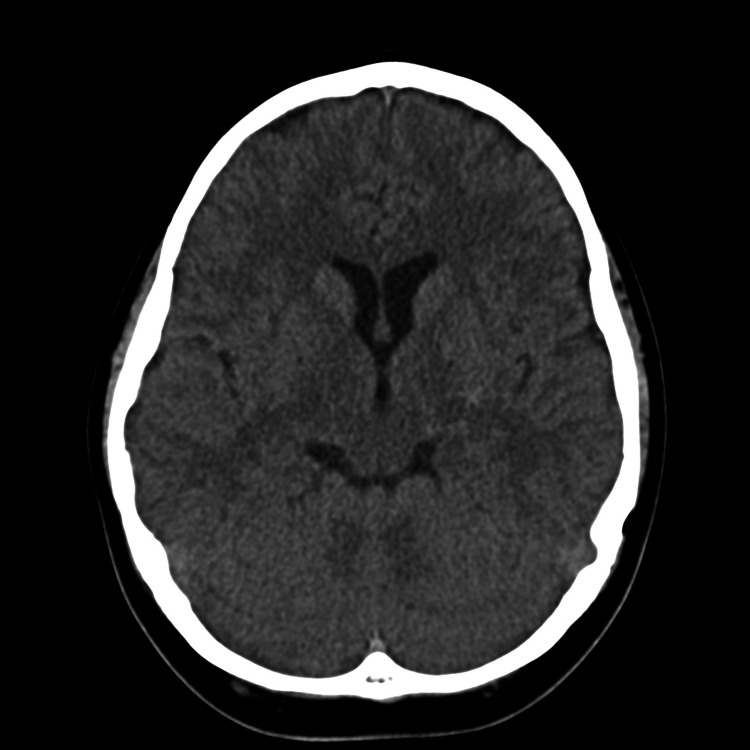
NECT of the brain showing mild density in the region of the left basal ganglia at the posterior limb of the internal capsule. A slight asymmetry of the lateral ventricles with the larger left lateral ventricle indicates possible cerebral hemiatrophy. No mass effect or hydrocephalus can be seen. NECT: non-enhanced computed tomography
